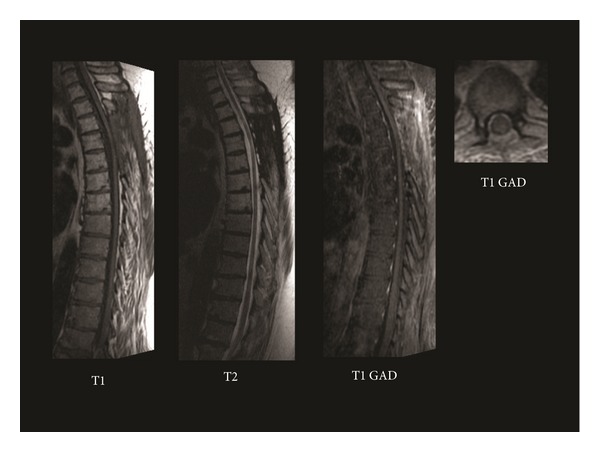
Postoperative MRI at 1-year followup shows 3 sagittal sequences (T1, T2, and T1 with gadolinium) on the left and an axial (T1 with gadolinium) on the right demonstrating no evidence of tumor recurrence.
